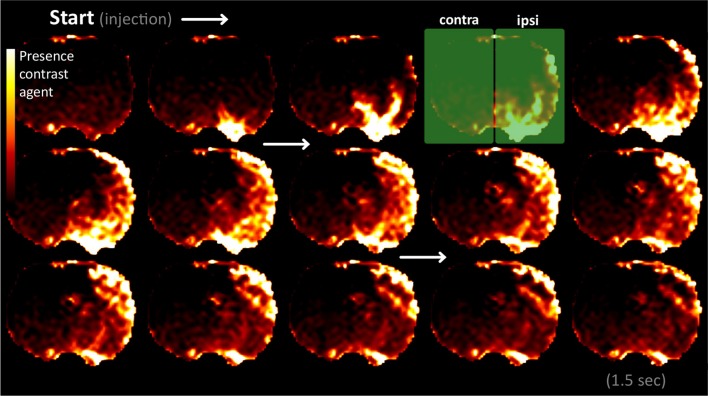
Perfusion MRI frames, demonstrating clear unilateral delivery of the contrast agent in the hemisphere ipsilateral to the side of cannulation. The frames were acquired at 100 ms intervals from the start of bolus administration until 1.5 s later (the temporal frame sequence runs from top left to bottom right). The frames are in coronal plane at 1 mm from bregma. Presence of contrast agent is displayed using a color scale: dark for low contrast concentrations and bright for high contrast concentration (in arbitrary units). The green rectangles mark the ipsi- and contralateral hemispheres.
